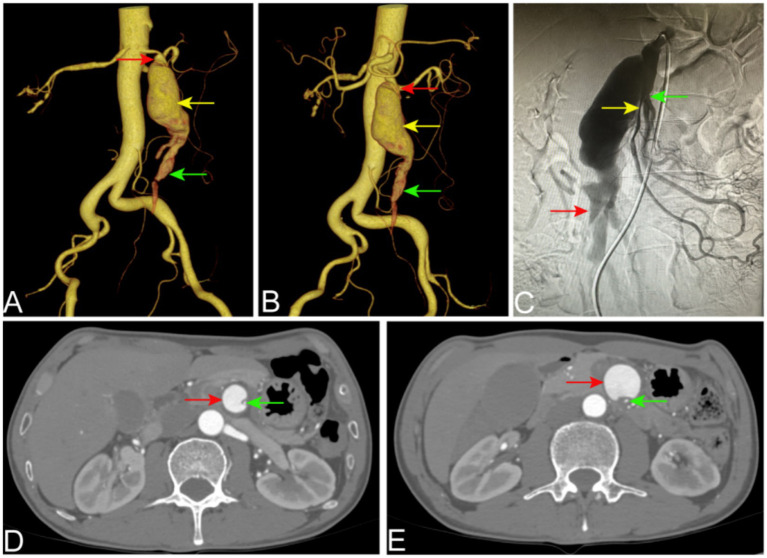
Preoperative imaging findings. (A,B) Emergency computed tomography angiography (CTA) demonstrated a dissecting aneurysm of the superior mesenteric artery measuring 4.2 × 4.8 cm (yellow arrow). The dissection involved the first proximal branch of the SMA (red arrow) and the distal SMA branches were supplied by the false lumen (green arrow). (C) Emergency angiography revealed a giant dissecting aneurysm of the superior mesenteric artery. The dissection involved the first proximal branch of the SMA (green arrow) as well as the distal SMA branches (red arrow) and the true lumen was compressed by the false lumen, appearing as a slit-like lumen (yellow arrow). (D,E) Axial CT images showed that the true lumen (green arrow) was nearly completely compressed and occluded by the false lumen (red arrow).
